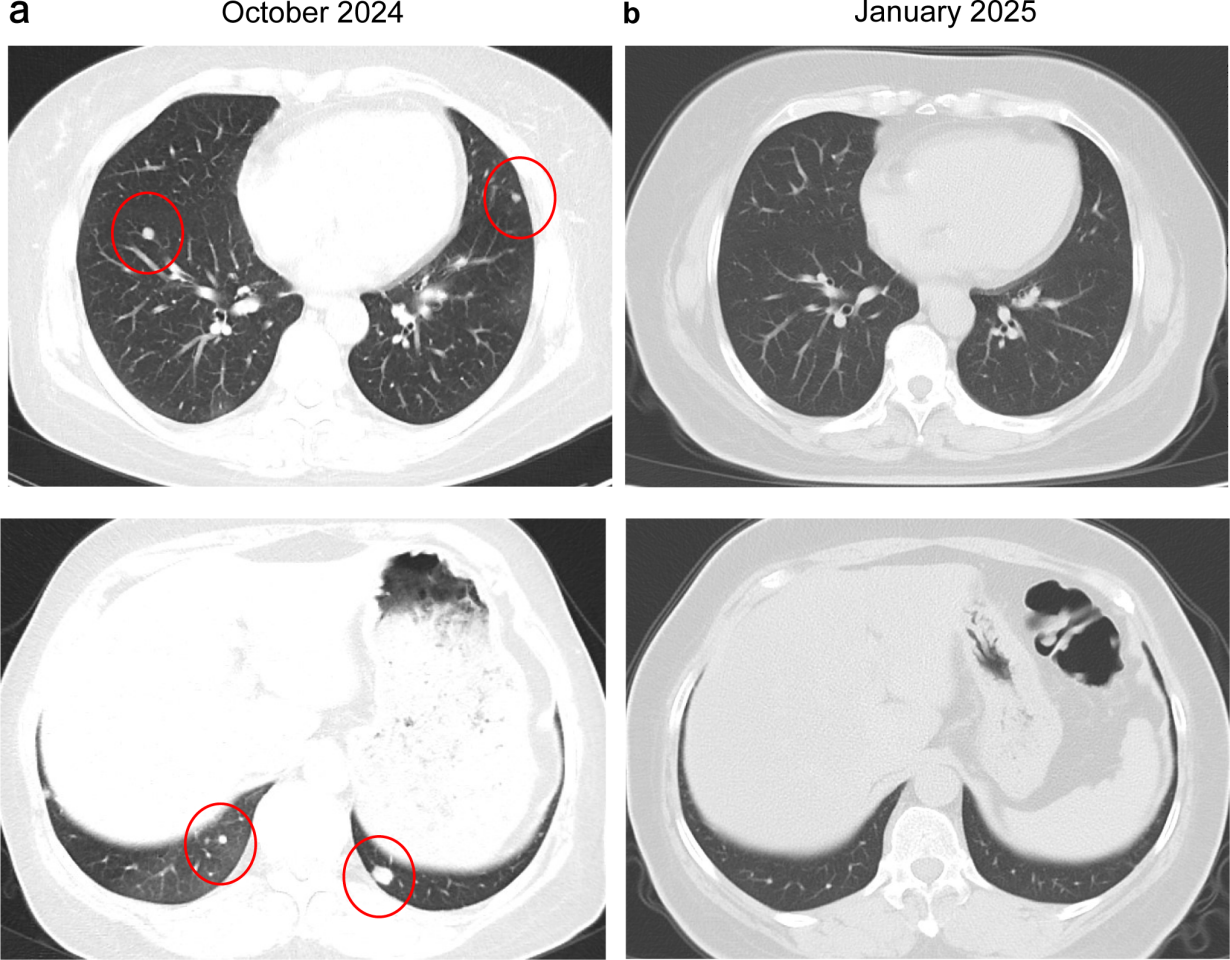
Chest CT scans before and after first-line chemotherapy. (a) Pre-chemotherapy CT image (October 25, 2024) demonstrates multiple bilateral pulmonary metastases. (b) Post-chemotherapy CT scan (January 13, 2025) shows significant remission of the pulmonary metastases. CT, computed tomography.
